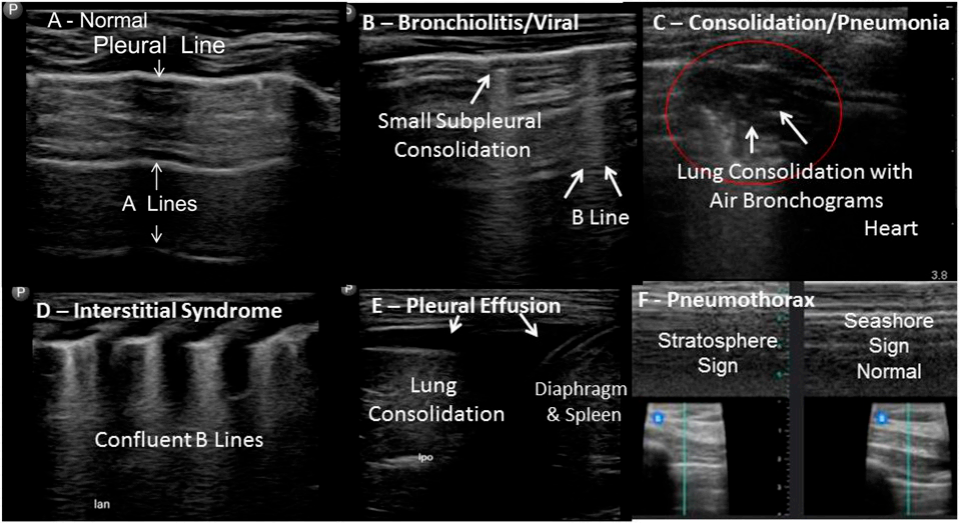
Examples of ultrasound findings. Interstitial syndrome refers to acute respiratory distress syndrome or severe viral LRTI, less commonly pulmonary edema.^15^
